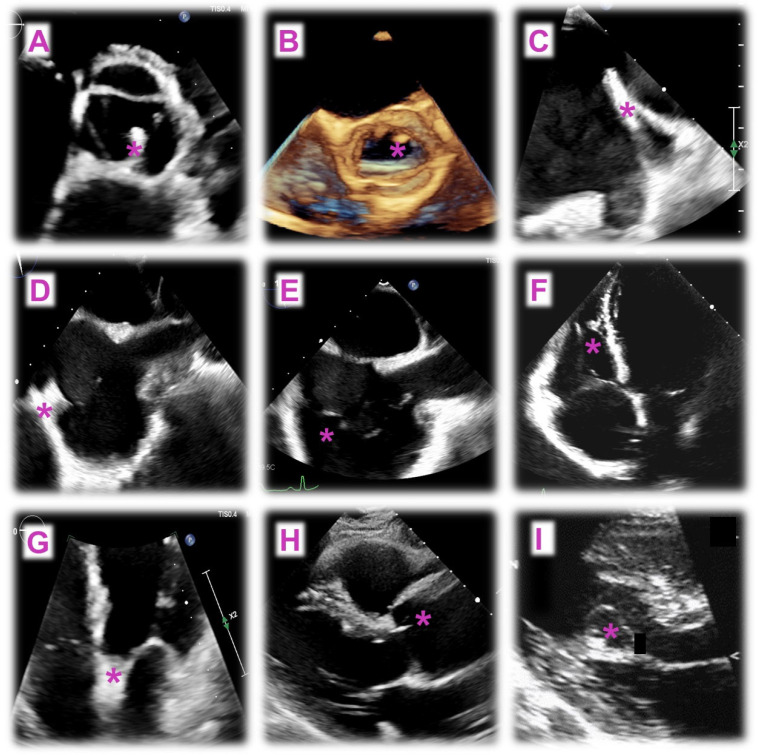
Echocardiographic images of masses and pseudomasses (*). Panel (A,B): Papillary fibroelastoma on TEE (A) and RT3DE (B); Panel (C): Coumadin ridge on TEE; Panel (D): Crista terminalis on TEE; Panel (E): Chiari network on TEE; Panel (F): Moderator band on TTE; Panel (G): Lipomatous hypertrophy of the interatrial septum on TEE; Panels (H): Lambl’s excrescences on a long-axis parasternal view, TTE; Panels (I): Cardiac blood cyst on TTE.
